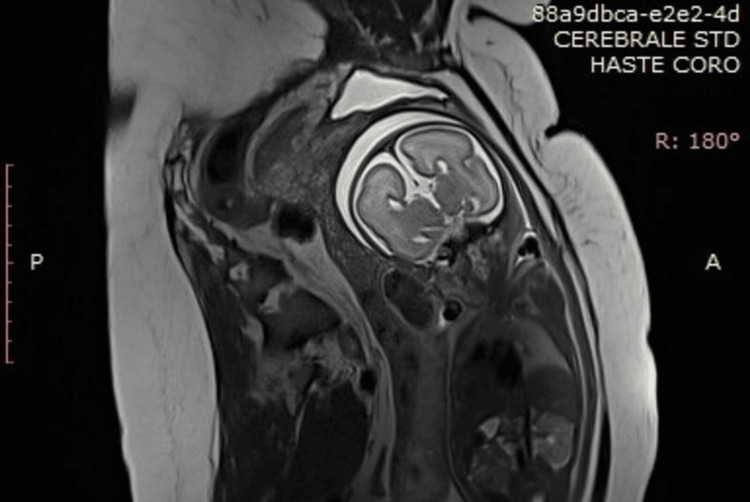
Fetal MRI in coronal T2 sequence showing the widening of the frontal lobes exhibiting a “bull’s horn” configuration
